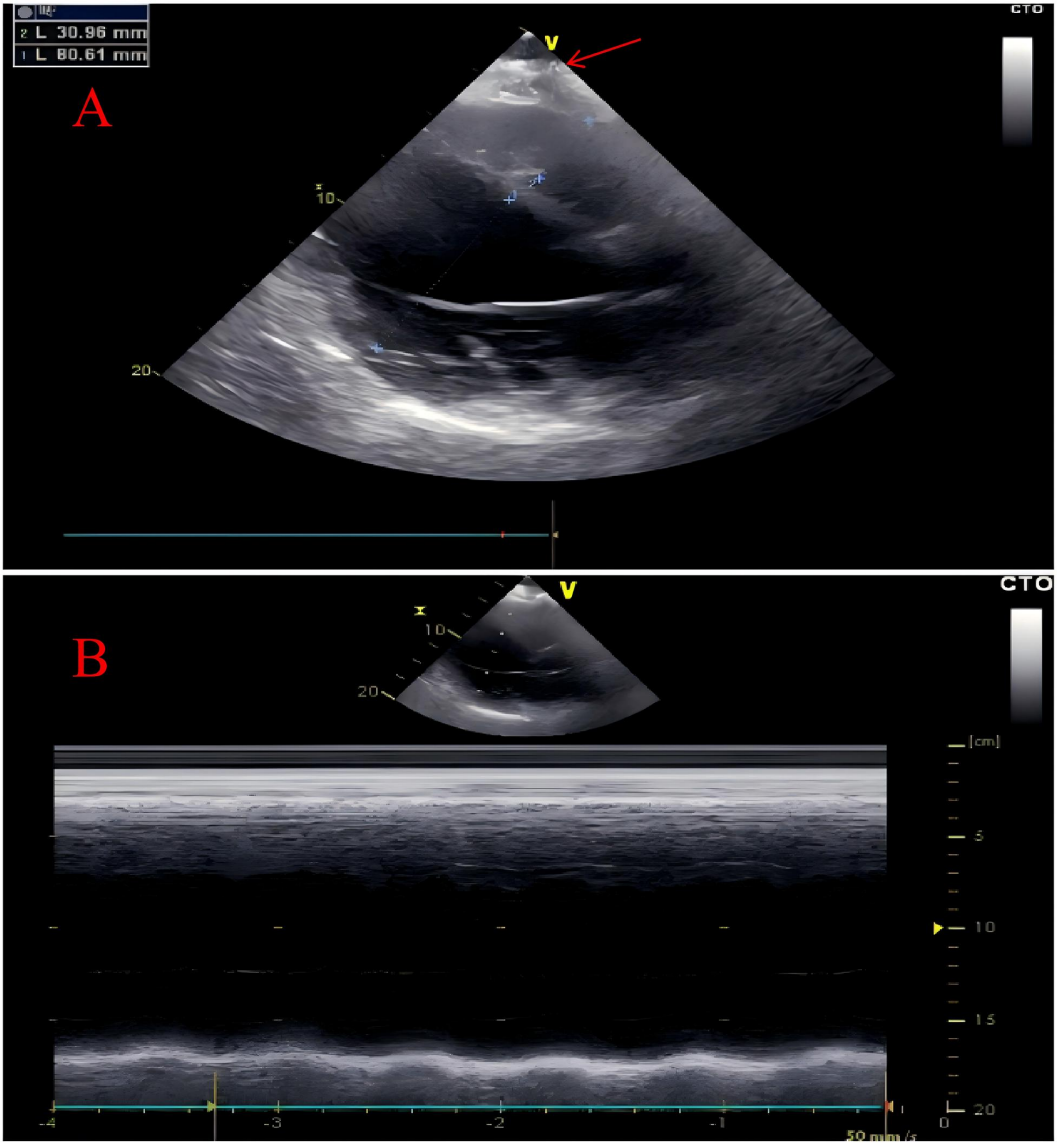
Transthoracic echocardiography. The transthoracic echocardiography revealed an overall enlargement of the heart with calcification at the cardiac apex (marked by red arrow) (A) Additionally, the left ventricle demonstrated impaired systolic function (B).
